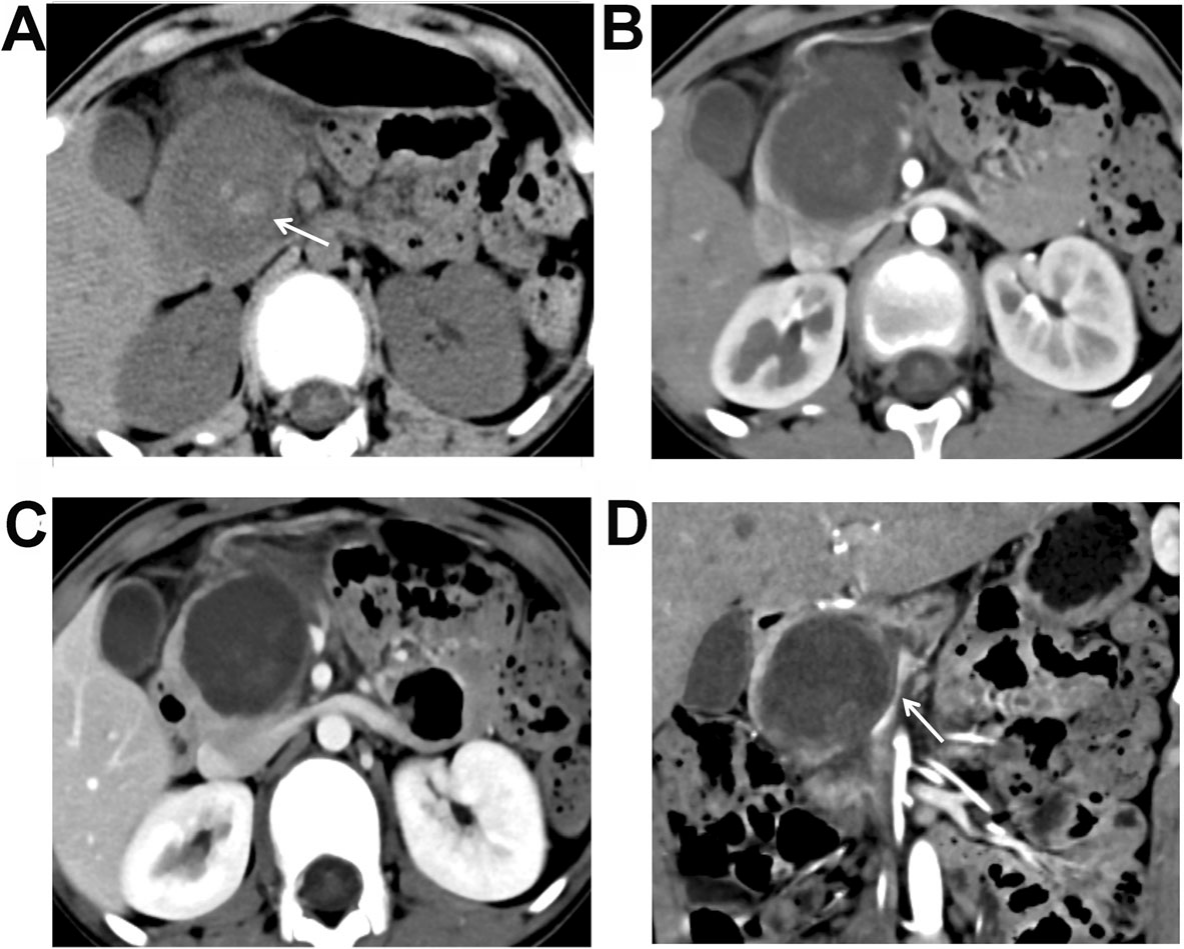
A 12-year-old female patient with vomiting and upper abdominal pain. Plain CT scanning + enhanced scanning showed pseudo-papillary neoplasm of pancreas (SPNP). a Plain scanning showed a huge oval cystic mass at the pancreatic head, and the boundaries were clear. Small patchy bleeding focuses were found in the mass (white arrow). b Low enhancement of the lesion was found in the arterial phase, which was lower than the normal pancreas. c A little enhancement of the cystic components was found in the portal venous phase, which showed “floating cloud” sign. d Reconstructed coronal image showed compressing and circuity of the superior mesenteric vein (white arrow)
